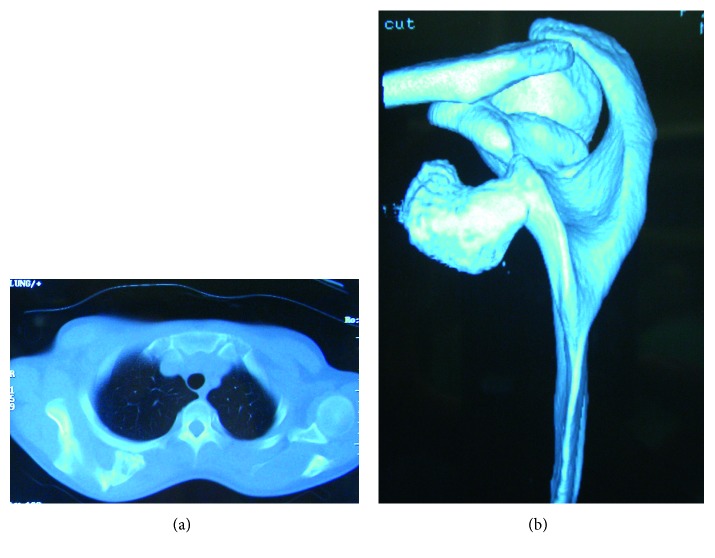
Preoperative computed tomography (CT) findings. (a) CT showed the mushroom-shaped osseous tumor composed of a cortex continuous with the scapular cortex, with a broad flat distal end that almost contacted the third rib. (b) Three-dimensional CT revealed the close relationship between the base of the osseous tumor and the superomedial scapular angle.
